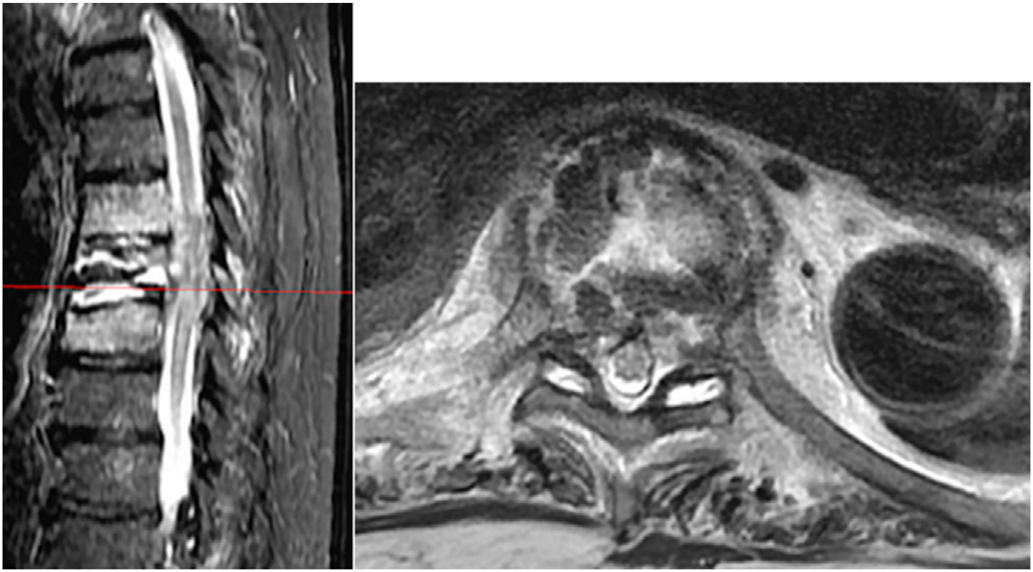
Magnetic resonance imaging (MRI) STIR of the thoracic and lumbar spine revealed abnormal T2 intramedullary hyperintensity spanning the T7-9 level with moderate to severe central canal stenosis and a 5 mm retropulsion of the T8 bone fragments disrupting posterior vertebral rim.
